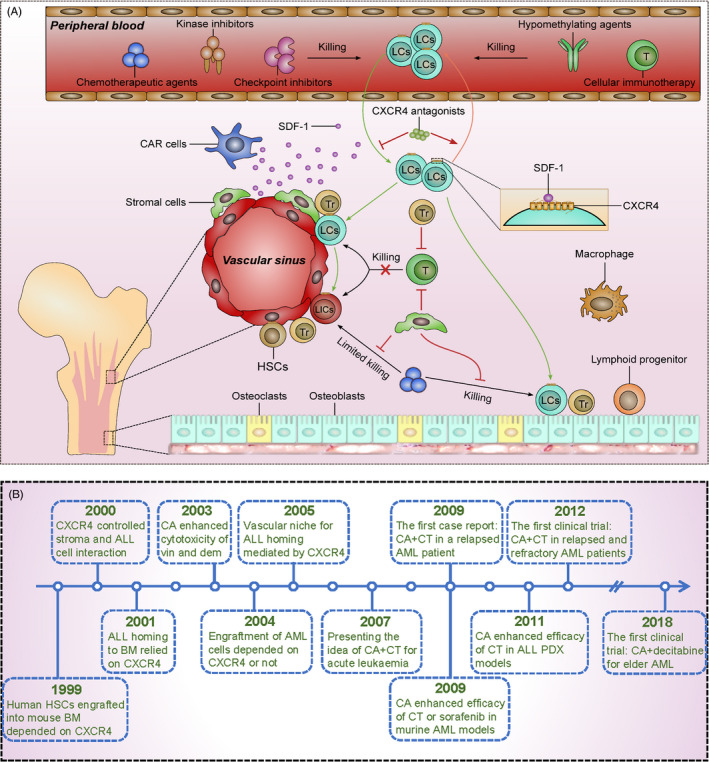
The major progress of research on SDF‐1/CXCR4 in AL. A, SDF‐1/CXCR4 plays important roles in homing and retention of leukaemia cells (LCs) in the BM. SDF‐1 produced by CXCL12‐abundant reticular (CAR) cells and stromal cells can recruit LCs into BM where they reside in special niches. Some LCs reside as leukaemia‐initiating cells (LICs). In the BM microenvironment, Tregs (Tr) and stromal cells protected LCs from killing by effector T cells (T) or chemotherapeutic agents. CXCR4 blockade with special antagonists can mobilize LCs from the protective microenvironment into peripheral blood and render them eradicated by chemotherapeutic agents, kinase inhibitors, checkpoint inhibitors, hypomethylating agents and cellular immunotherapy. B, Shown is the major progress of SDF‐1/CXCR4 function and the potential of CXCR4 as a therapeutic target in AL during the last three decades. CA: CXCR4 antagonists; vin: vincristine; dem: dexamethasone; CT: chemotherapy
